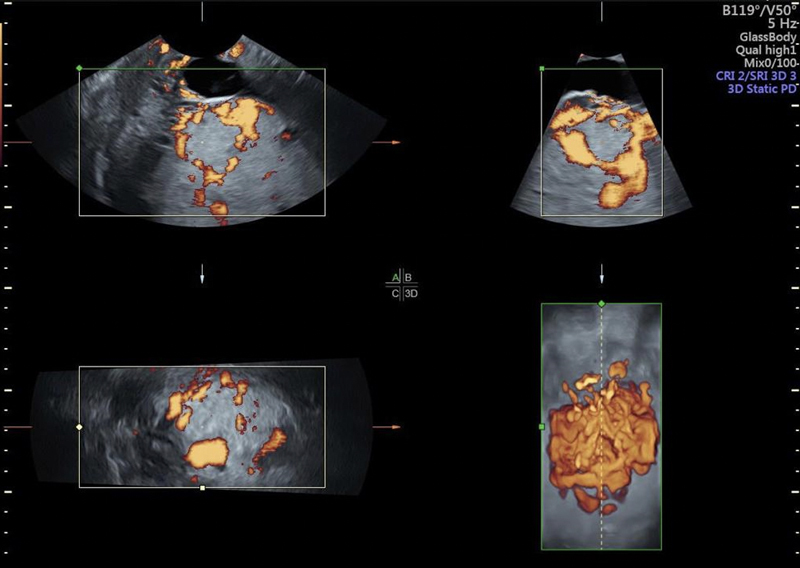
Multiplanar 3D representation of the placenta and uterovesical interface associated with power Doppler, showing uterovesical and intraplacental hypervascularity
